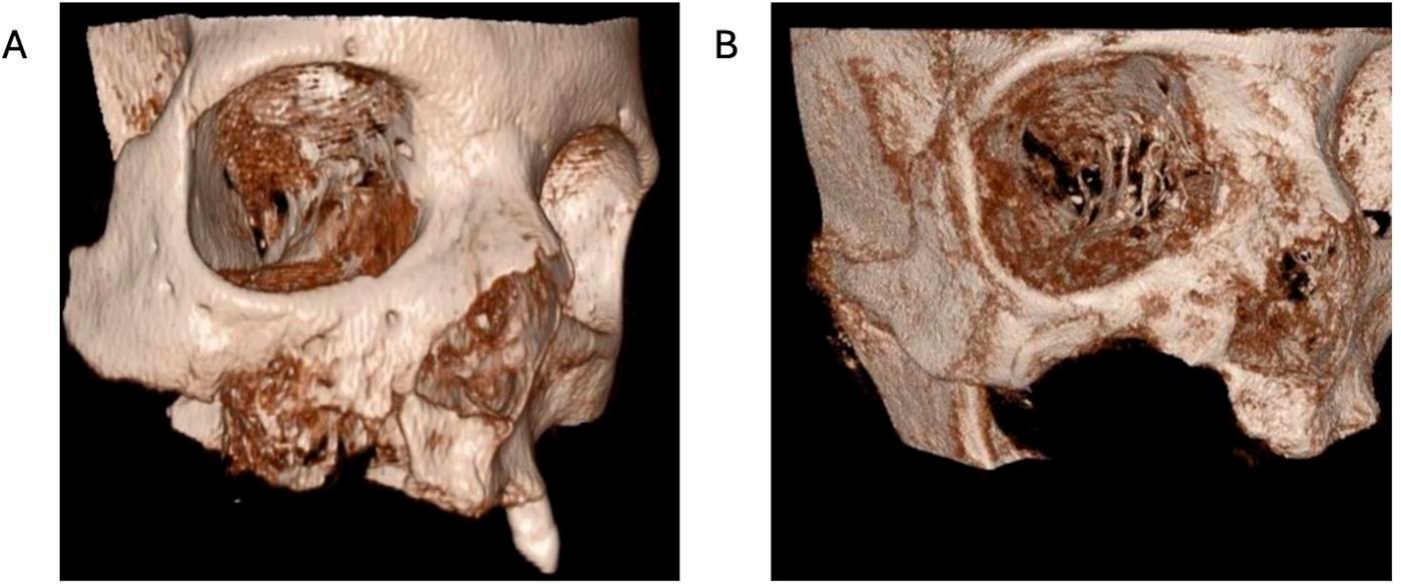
CBCT image. (A) Before surgical treatment (B) After 9 months.
